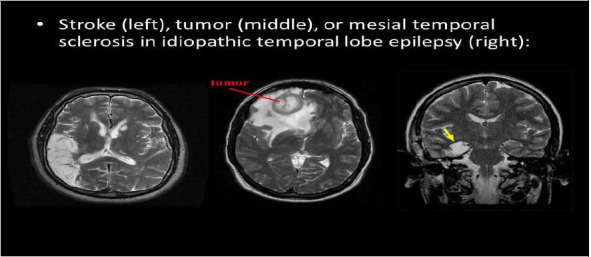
Abnormal brain MRI in epilepsy.
